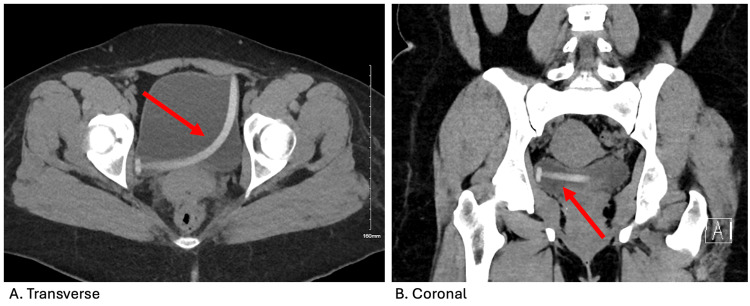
(A and B) CT scans (transverse and coronal views) of the foreign body located in the bladder, indicated with a red arrow.
